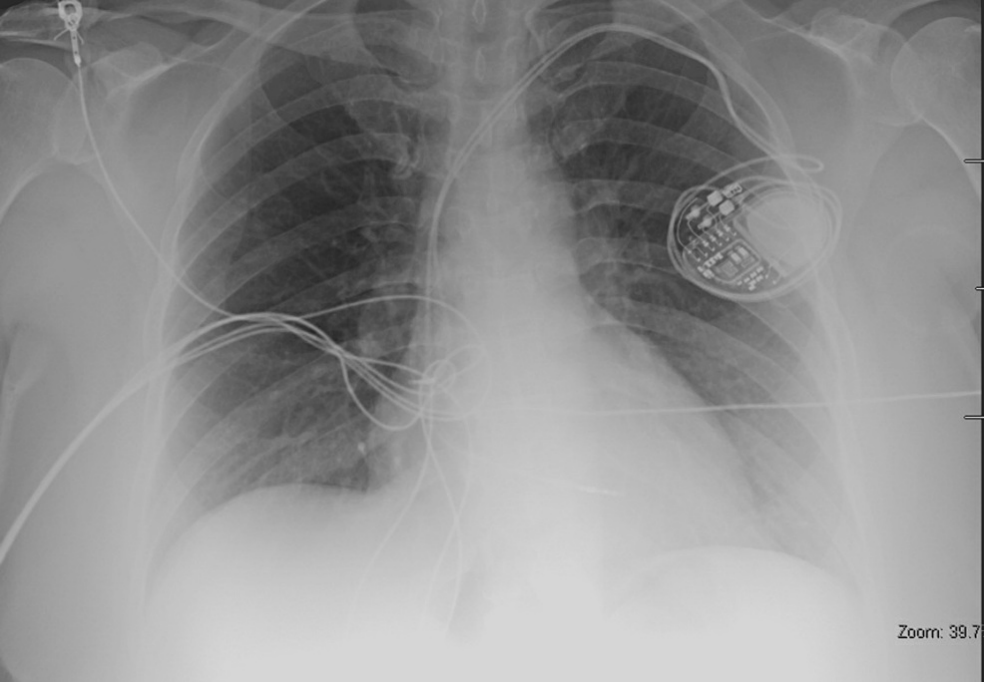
Chest radiograph demonstrating adequate cardiac pacemaker placement
